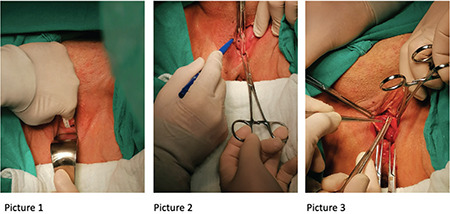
Picture 1, 2. The hyperintense focus marked on the vaginal wall using MRI mapping and a full-thickness linear incision from the urethrovesical junction and extending the cervico-vesical junction Picture 3. Taken of a biopsy of 0.5x0.5 cm from the region marked by MRIM
